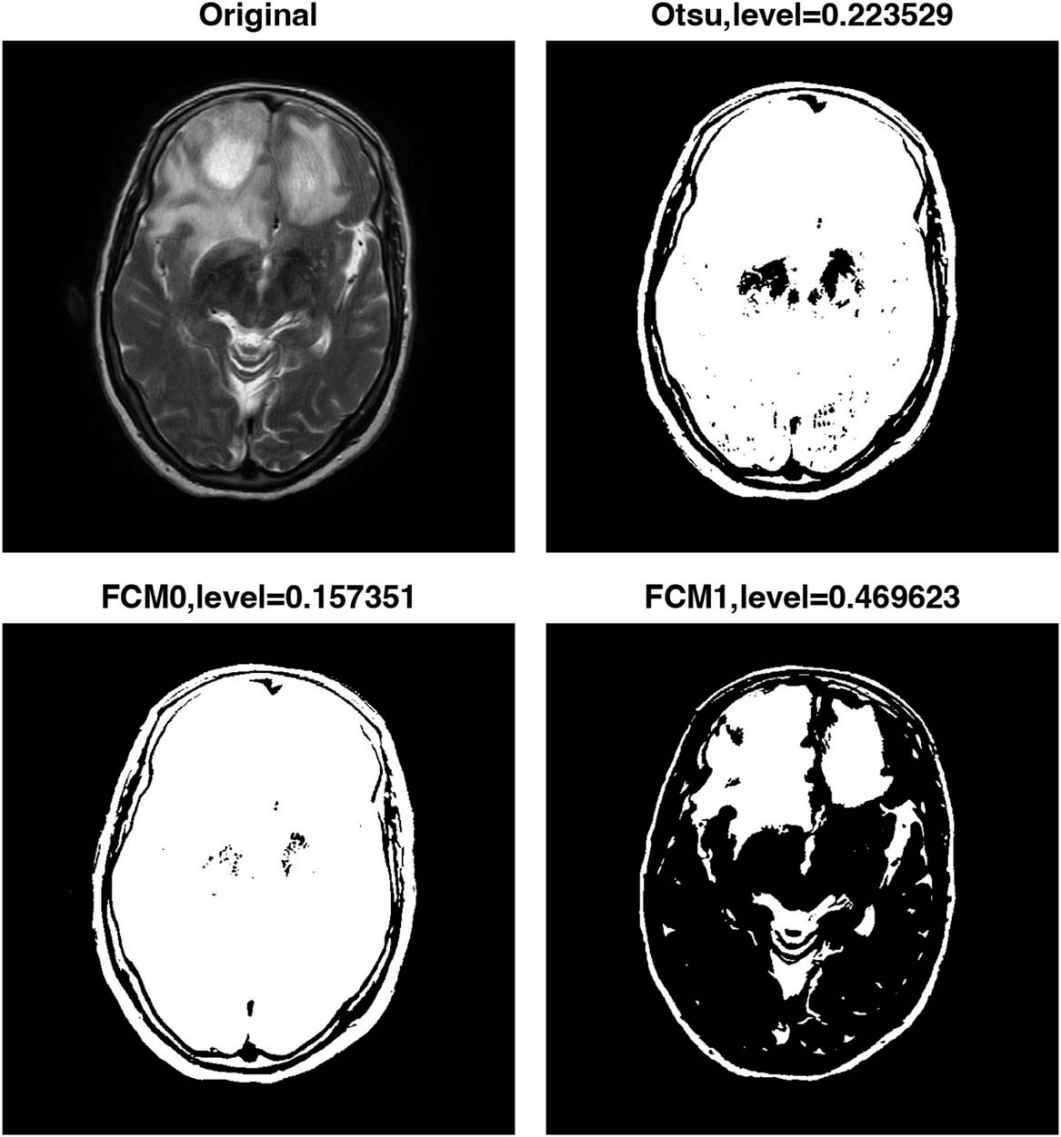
Pre-processing steps for segmentation of multi-focal glioblastoma multiforme tumours in axial T2-weighted MRI images of patient 1. FCM, fuzzy c-means.
